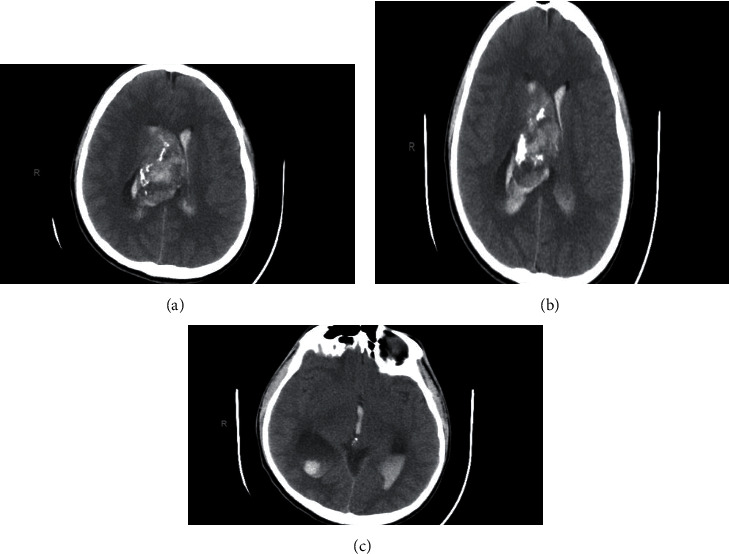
Head CT axial view without contrast.
